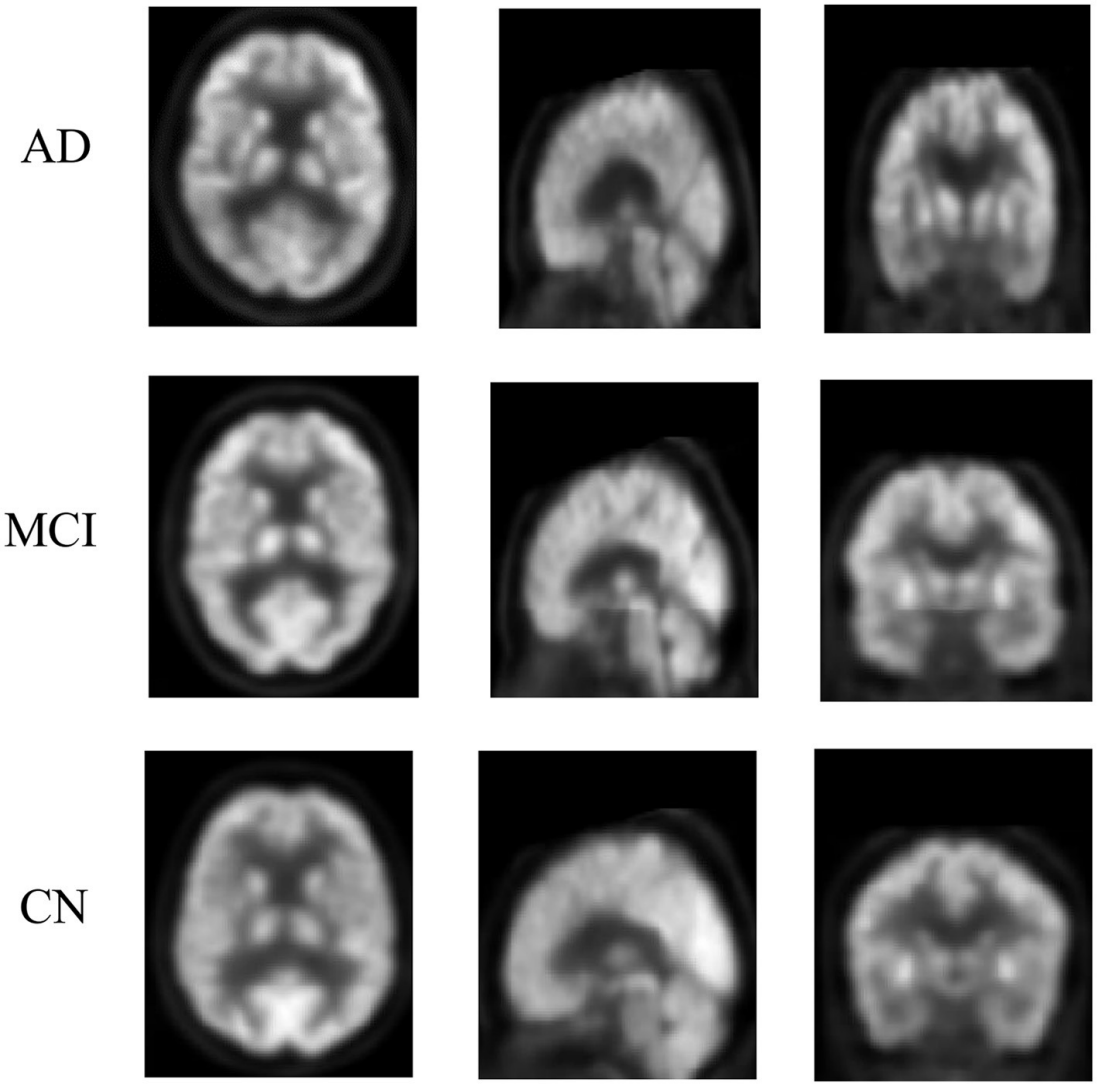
Example of FDG-PET imaging from ADNI. Each row represents a PET imaging slice on a three-plane view. The three rows are: a 73-year-old man with AD, an 81-year-old woman with MCI, and a 71-year-old man without MCI/AD. The difference between them is difficult to identify with the naked eye.
